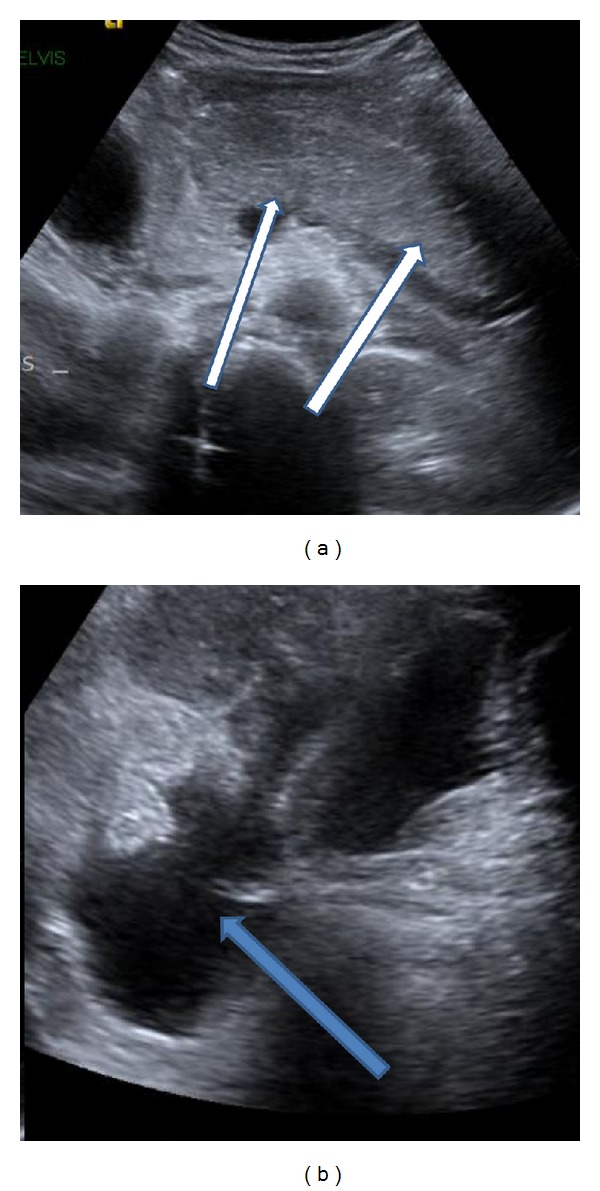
(a) Ultrasound of epigastrium region showing edematous pancreas (white arrows) and (b) ultrasound of lower abdomen showing free fluid (blue arrow).
